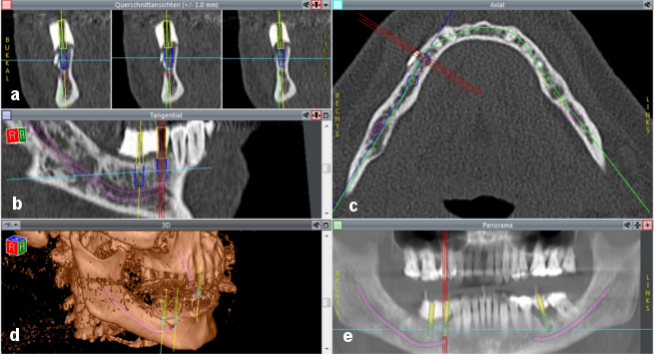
Screenshot demonstrating implant planning a) coronal view; b) sagittal view; c) axial view; d) 3D view; e) pseudo-panoramic radiograph. The purple line demonstrates the inferior alveolar nerve. The blue cylinders represent the implants selected from the implant library. The yellow cylinders show the virtual abutments, which allow the implants to be placed in relation to the planned positions of the abutments. This allows an adequate prosthetic restoration to be achieved on the basis of the principle of backwards planning. Once the set-up was completed, the scan template was filled with barium sulfate. The planned location of the abutments was created in the template with a pilot drill. The green line (c) represents the panoramic curve, which allows a pseudo-panoramic radiograph to be generated. The yellow lines (a, b and d) show the vertical axis of the implant. The light blue lines show the horizontal axis of the implant. The three red lines (b, c and e) represent the axes of the implant that is being positioned.
